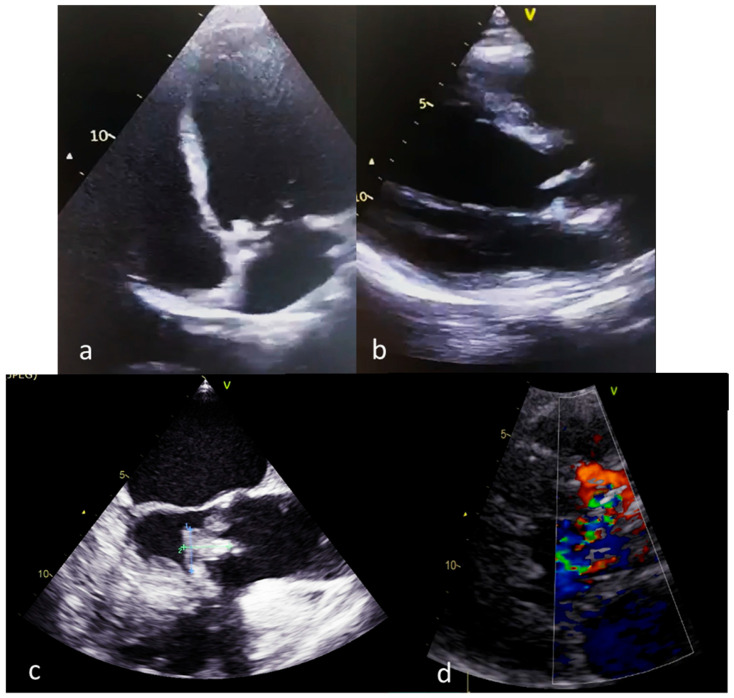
Echocardiographic findings in pregnant patients with IE (a,b): transthoracic echocardiography, aortic valve vegetations; (c): transesophageal echocardiography; (d): transthoracic echocardiography, aortic regurgitation secondary to aortic valve vegetation).
